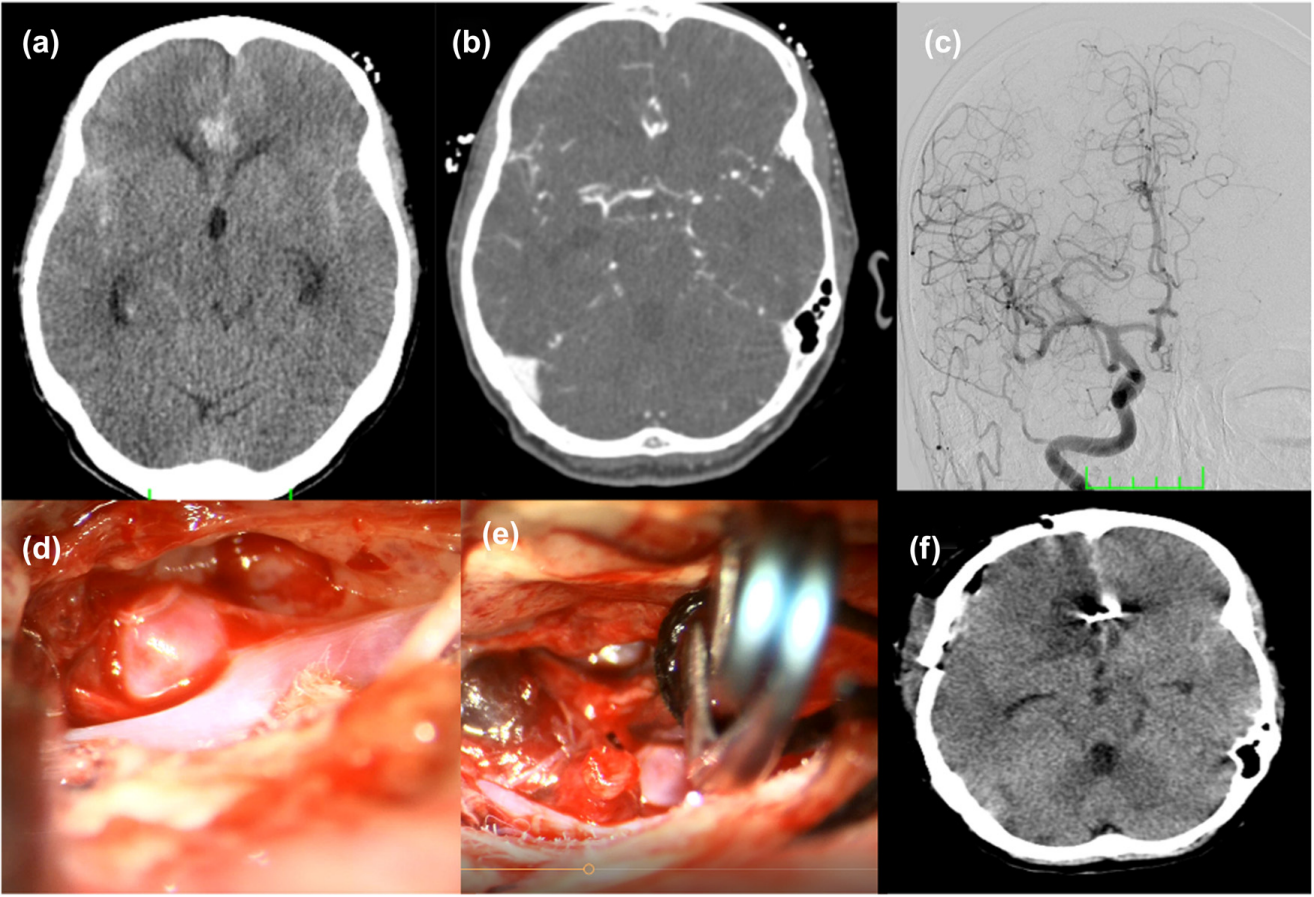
Representative images (a) Preoperative cranial CT: Fisher grade 2, (b) Preoperative Cranial CT angiography, (c) Preoperative DSA, (d) Intraoperative Acom Aneurysm, (e) Peroperative aneurysm clips, (f) Postoperative cranial CT.
